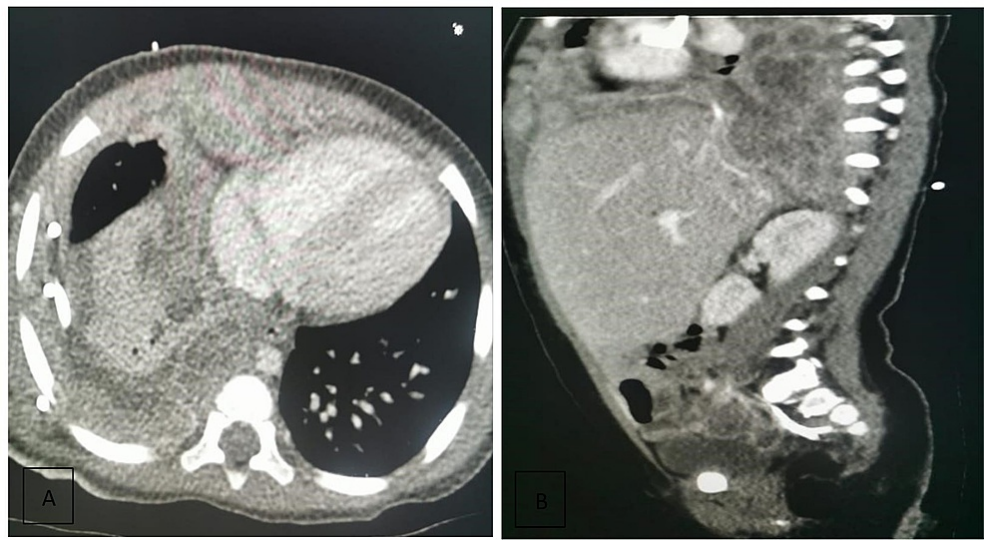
(A) Axial computerized tomography (CT) cut shows a lung base lobulated mass (7.3 x 6.4 x 4.4 cm). (B) A longitudinal CT cut shows the extension through the diaphragm.
